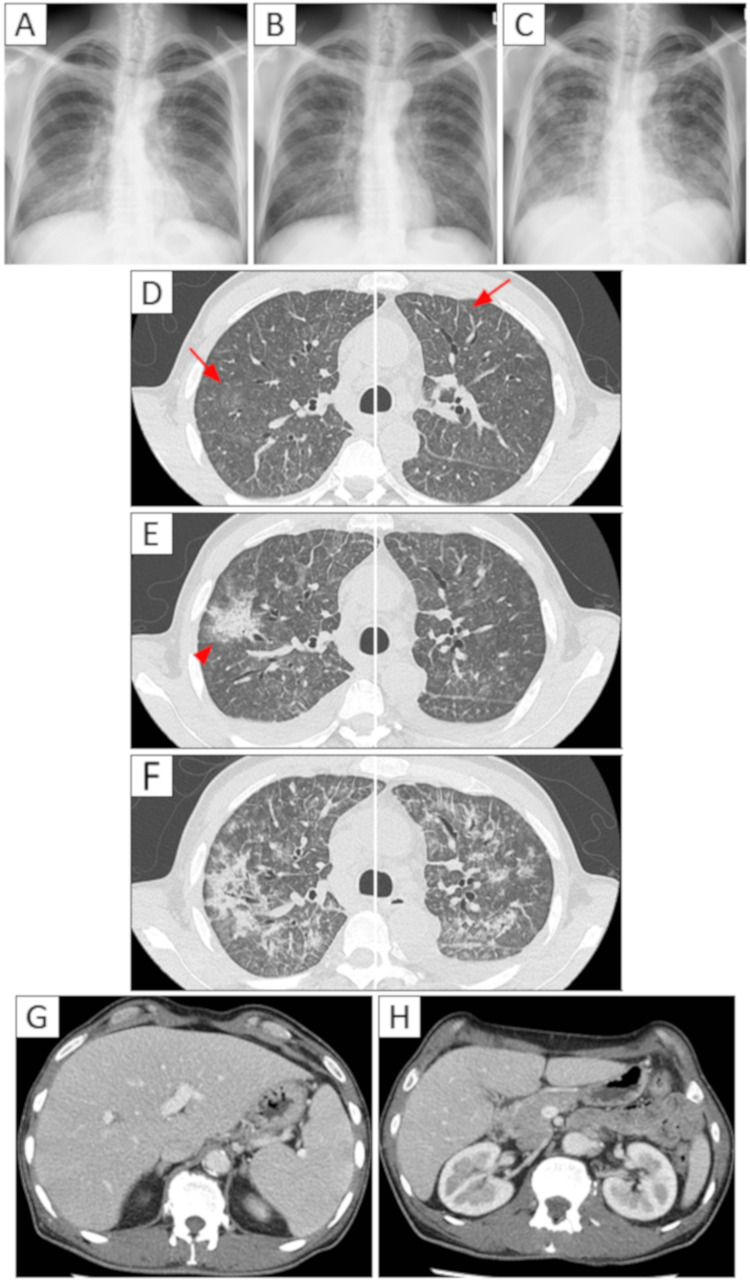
CXR and chest CT images on admission and after admission. CXR on admission showed diffuse ground-glass opacities in both lungs (A). After discharge from the hospital, CXR showed new infiltrative shadows in addition to diffuse ground-glass opacities in both lungs (B). Chest CT on admission showed diffuse ground-glass opacities in both lungs with thickening of the interlobular septal wall (red arrow) (D). The chest CT showed new infiltrative shadows in addition to diffuse ground-glass opacities in both lungs (red arrowhead) (E). CXR and chest CT before VATS lung biopsy showed further exacerbation of the previous imaging findings (C, F). Contrast-enhanced CT of the abdomen on admission, showing hepatosplenomegaly (G) and bilateral kidney enlargement (H).
